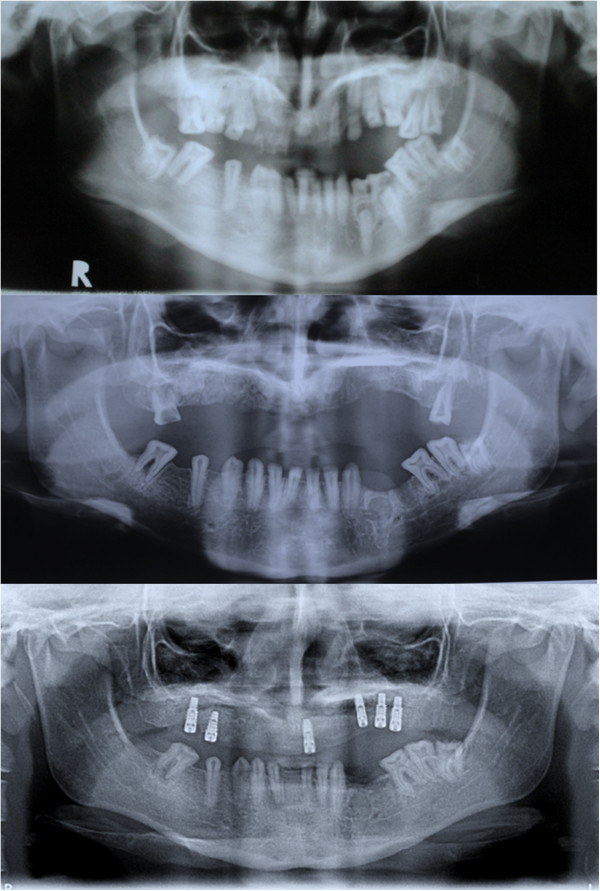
Initial radiography (top), post extraction (middle) and implants in situ.
